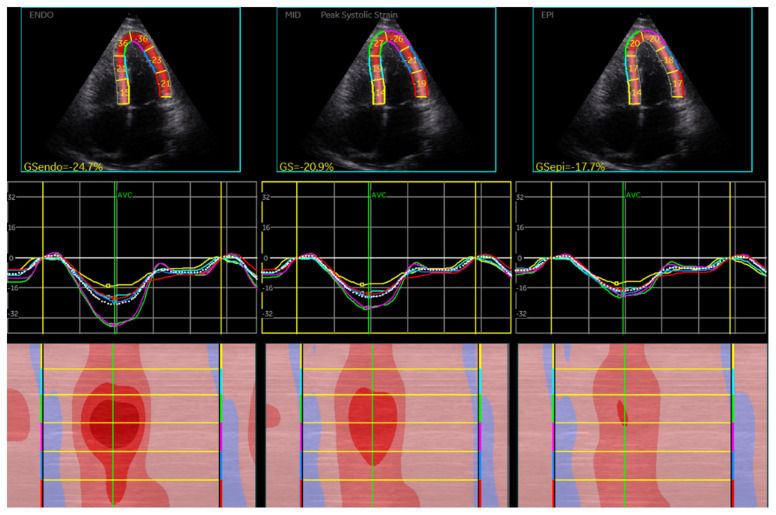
Example of left ventricular (LV) multilayer global longitudinal strain (GLS) in transthoracic echocardiography (TTE) four-chamber view.
